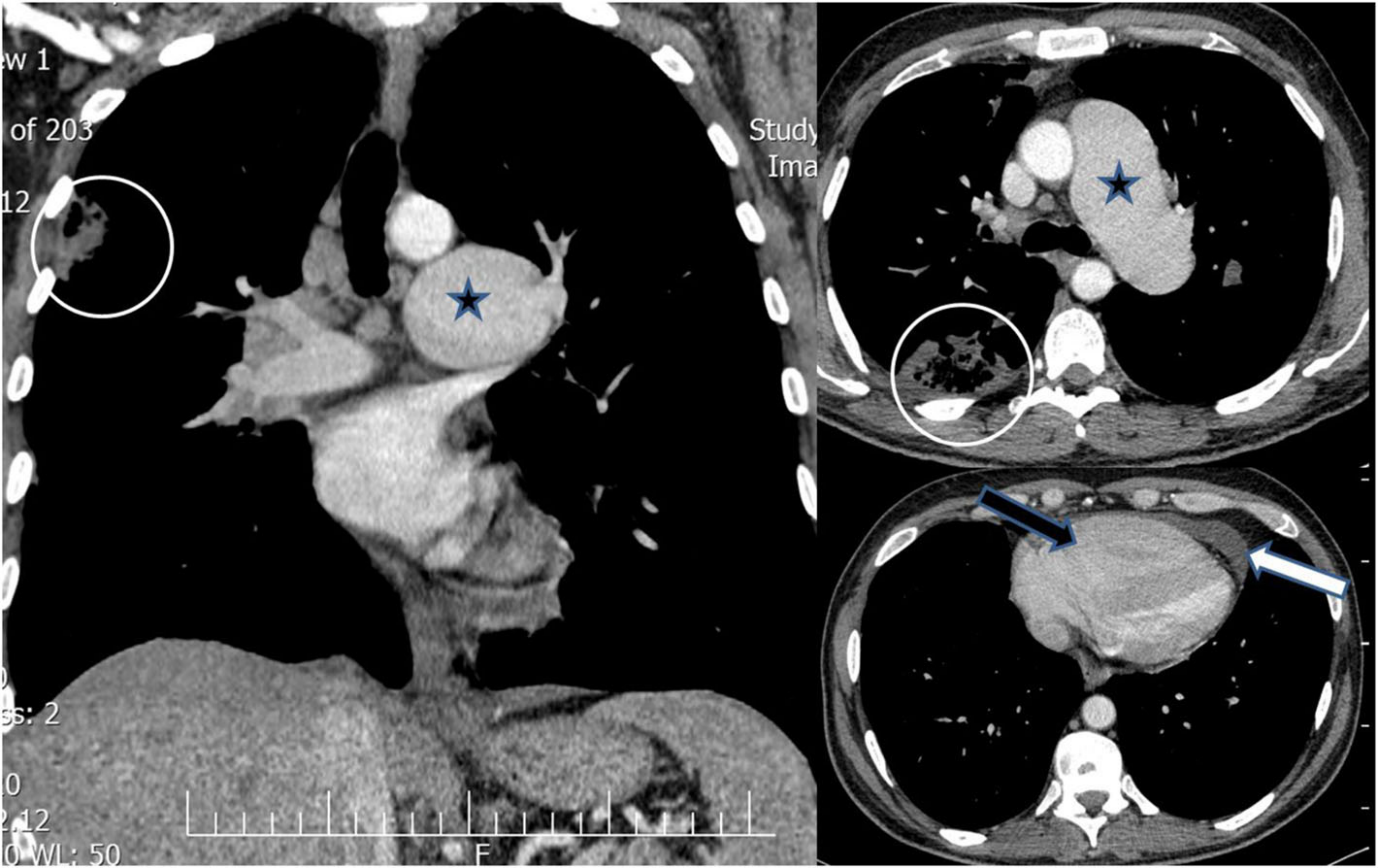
Axial and reconstructed coronal contrast-enhanced chest CT scan (mediastinal window): severe enlargement of main pulmonary artery and left pulmonary artery (black star) compatible post stenotic dilatation due to severe pulmonary stenosis(PS), large cavitary lesion (white circle) at RUL in favor of septic emboli,pericardial effusion (white arrow) and right ventricular enlargement (black arrow)
